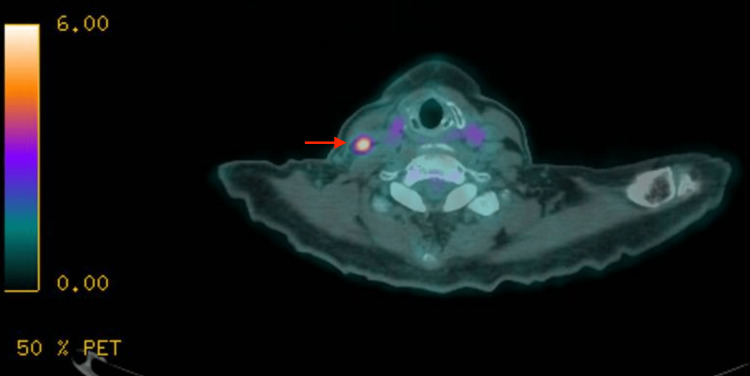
PET-CT depicting lymph node metastasis to the right at level IV, corresponding to a lymph node as seen on an MRI of the neck in Figure 5 CT, computed tomography; MRI, magnetic resonance imaging
